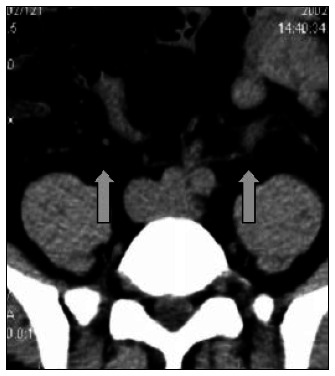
Collecting system dilatation. Noncontrast helical computed tomography (NCT) on a patient with a stone in the distal left ureter, showing ureteral dilatation in comparison with the contralateral normal side (arrows).
